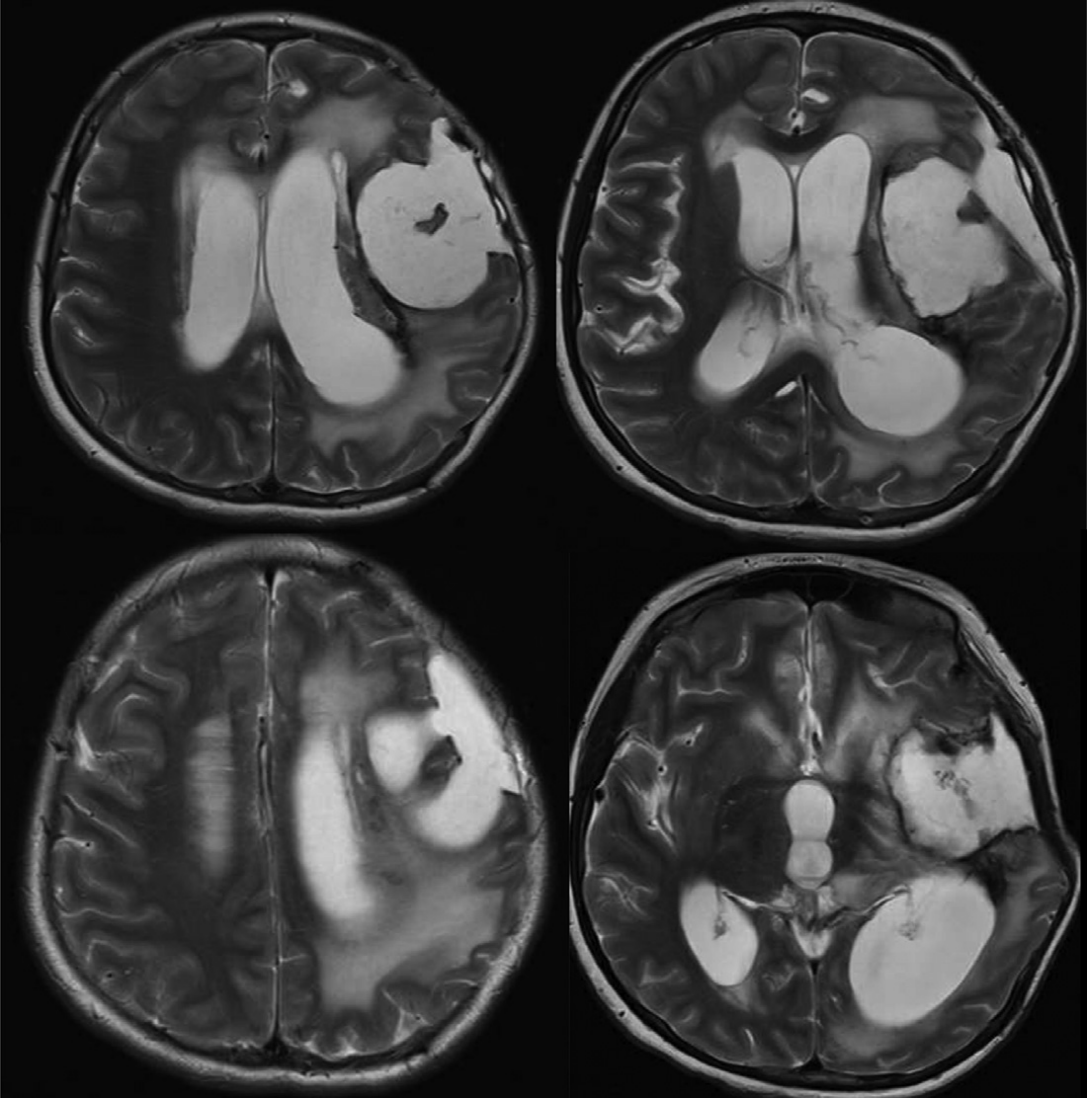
MRI brain (T2-weighted): Large left frontoparietal cystic lesion with mass effect and obstructive hydrocephalus. MRI of the brain (T2-weighted axial images) reveals a large, well-defined, hyperintense cystic lesion in the left frontoparietal region, causing significant mass effect with compression of the adjacent lateral ventricle and a mild midline shift. Associated vasogenic edema extends into the surrounding white matter, and moderate ventricular dilation suggests obstructive hydrocephalus. Postsurgical changes, including a craniotomy defect, are noted without evidence of acute hemorrhage.
